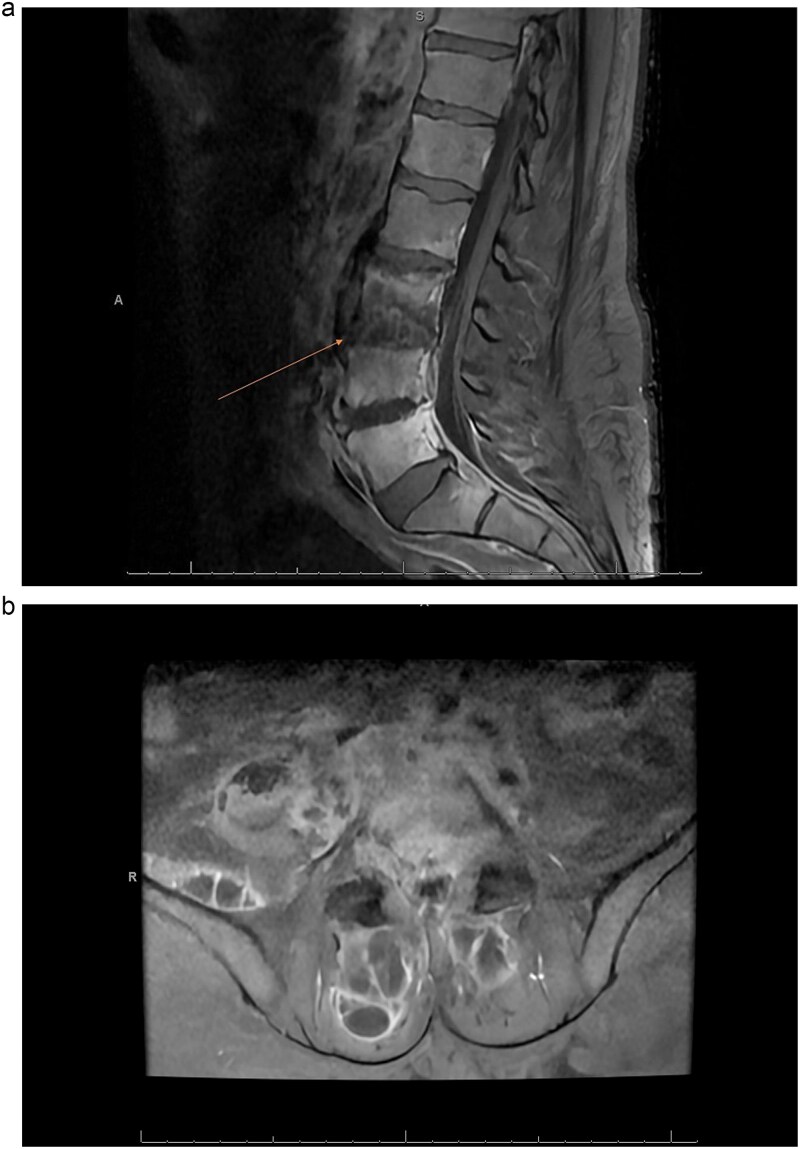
(a) MRI sagittal lumbar T1 with gadolinium contrast showing osteodiscitis at L3–4 and L4–5 vertebrae with epidural abscess. (b) Axial lumbar MRI T1 with gadolinium contrast showing epidural abscess with iliopsoas and paraspinal abscesses.
